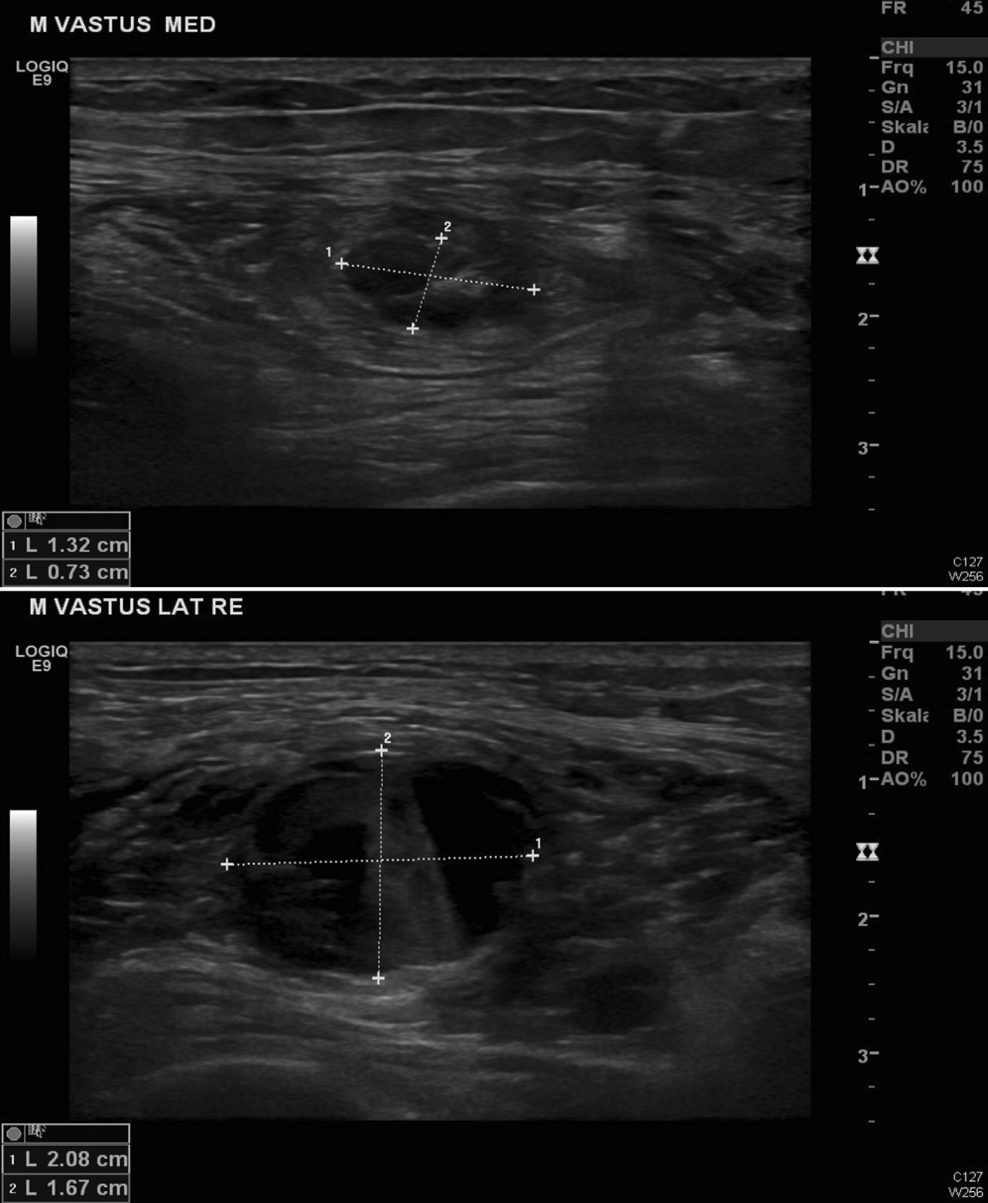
Sonographic evidence of an intramuscular hematoma: a) in the lateral vastus muscle (ca. 2 × 2 × 28 cm), and b) in the medial vastus muscle (ca. 1.5 × 1 × 1.5 cm) (Patient 1).
